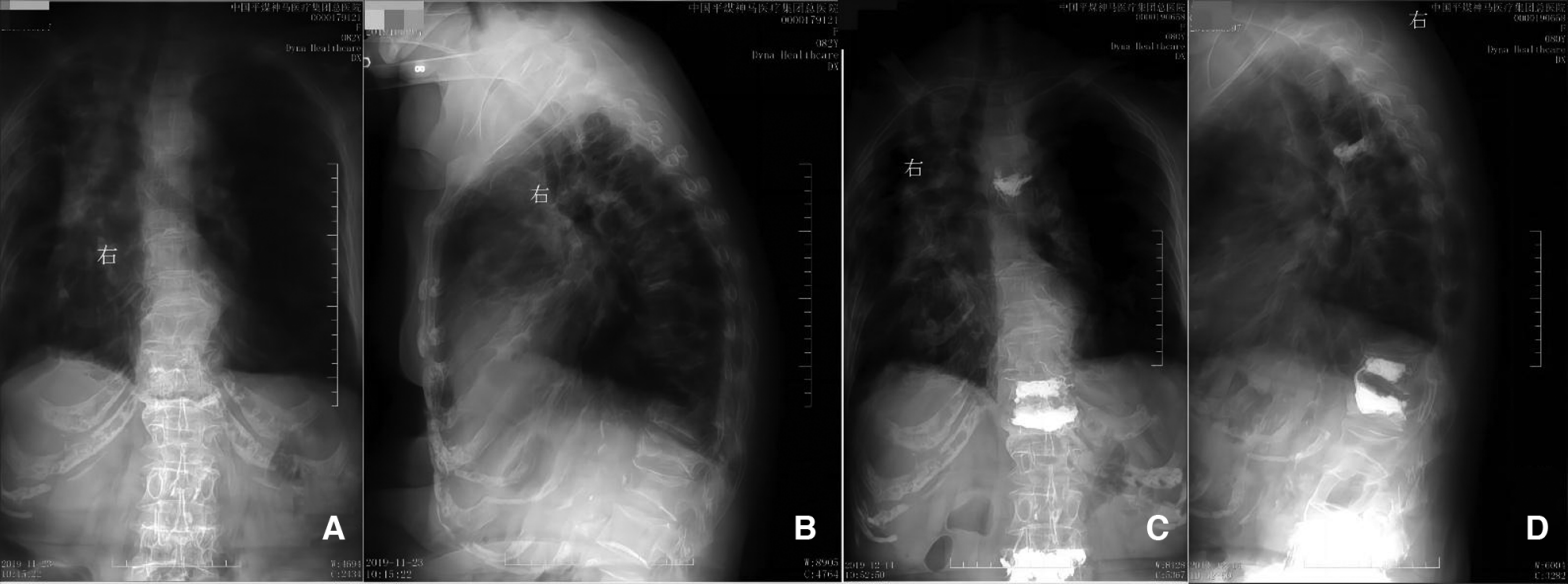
Preoperative (panels A and B) and postoperative (panels C and D) radiographs. Panels A and B correspond to the preoperative anterior and lateral positions, respectively, indicating compression changes in the 5th thoracic vertebra. Panels C and D correspond to the 12th day postoperatively demonstrating anterior and lateral positions respectively, showing the cemented 5th thoracic vertebra.
